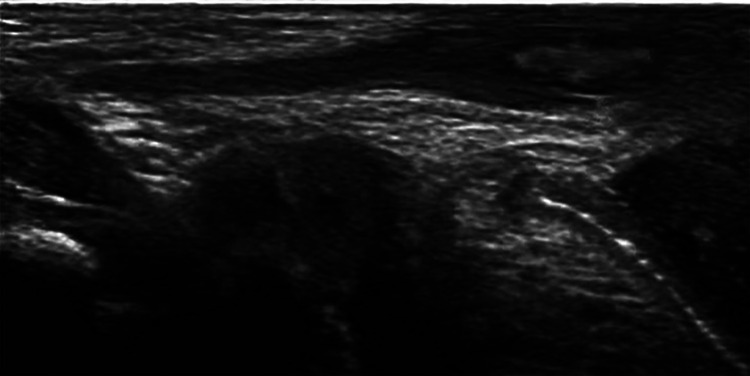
Ultrasound scan showing a hyperechoic nubbin located in the scrotum.
